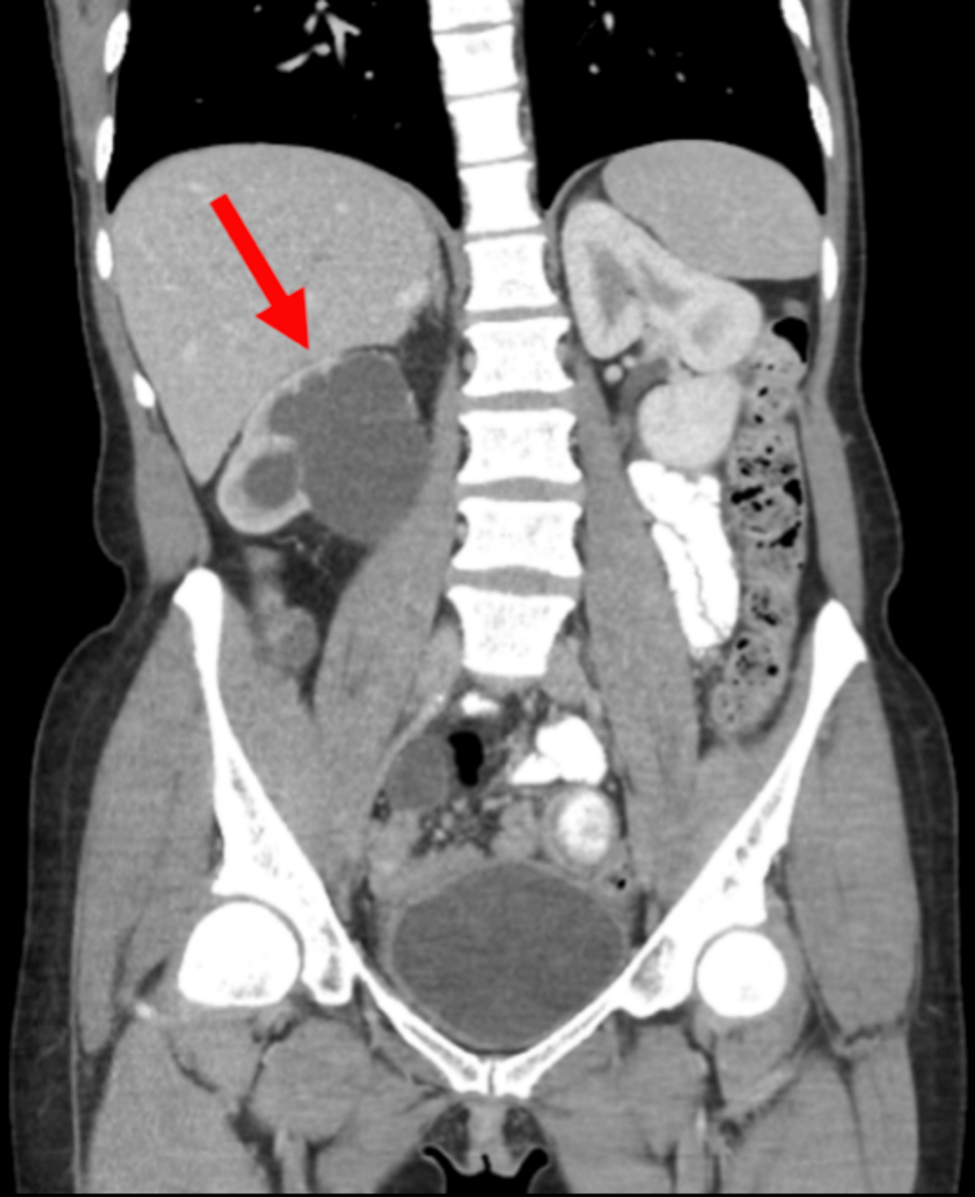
Severe hydronephrosis. The patient underwent PET-CT imaging with injection of 18F-fluorodeoxyglucose, which demonstrated severe hydronephrosis (arrow) of the right kidney. The ureter was diffusely dilated to just beyond the level of the iliac vasculature. The left kidney appeared normal. PET-CT, positron emission tomography-computed tomography
